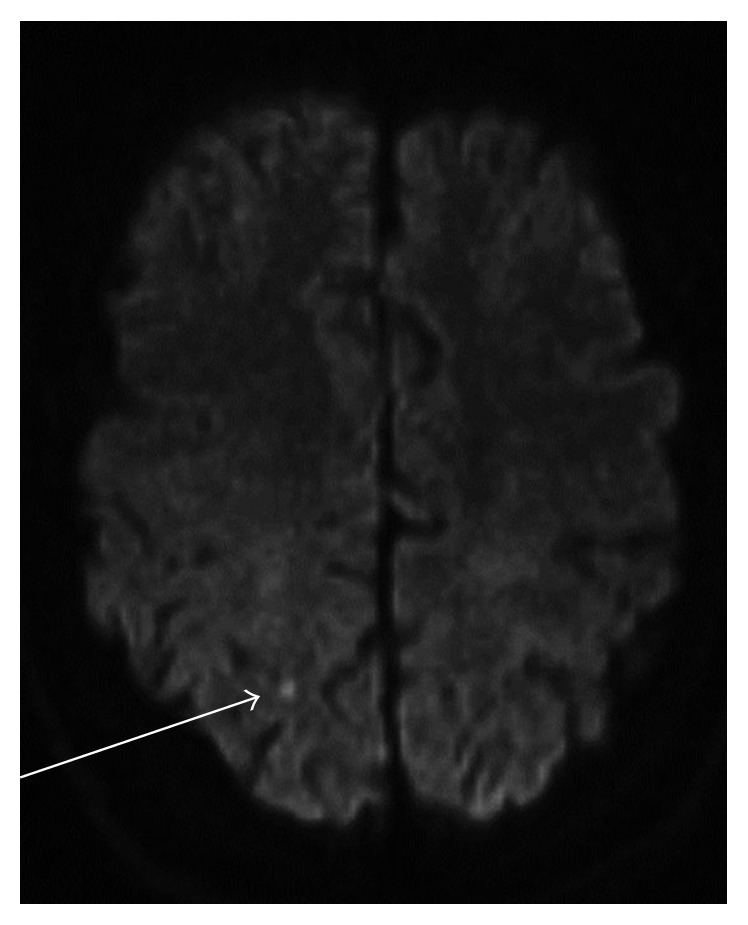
MRI brain demonstrating a focus of restricted diffusion in the right parietal lobe in keeping with acute infarction.
